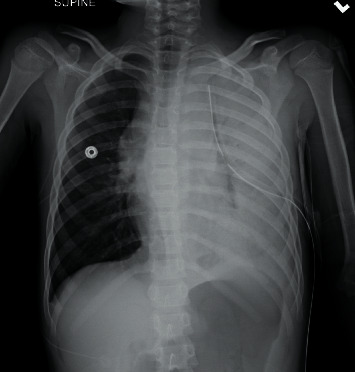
Immediate postchest tube thoracostomy chest radiograph demonstrating resolution of the mass effect and midline trachea.
